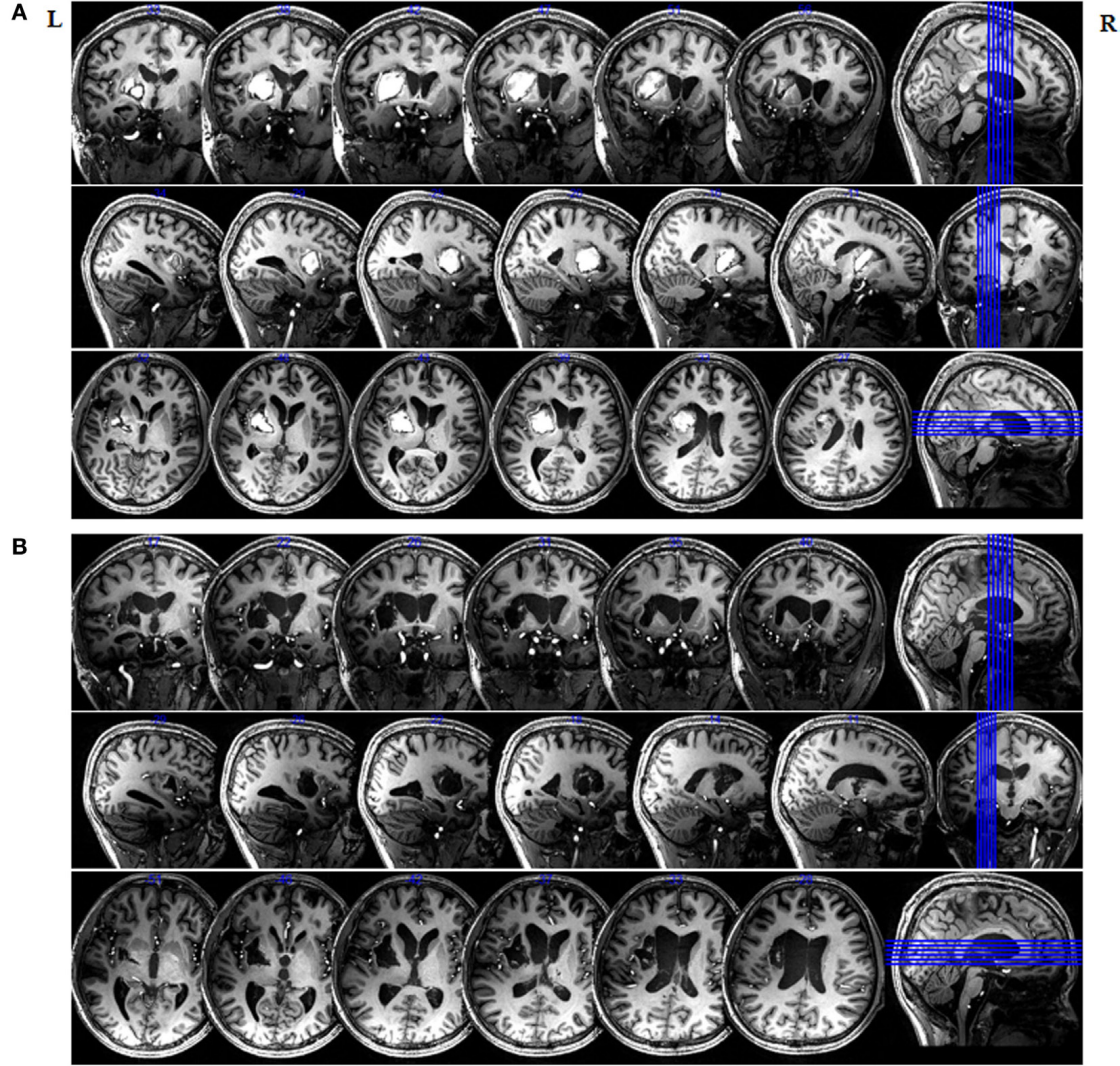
Magnetic resonance imaging (MRI) of the *in vivo* delineation of the patient’s entire brain at 7 T. 3D-T1-weighted sections of an MRI image of the entire brain obtained at (A) 1.5 months and (B) 5 months after initial injury.
